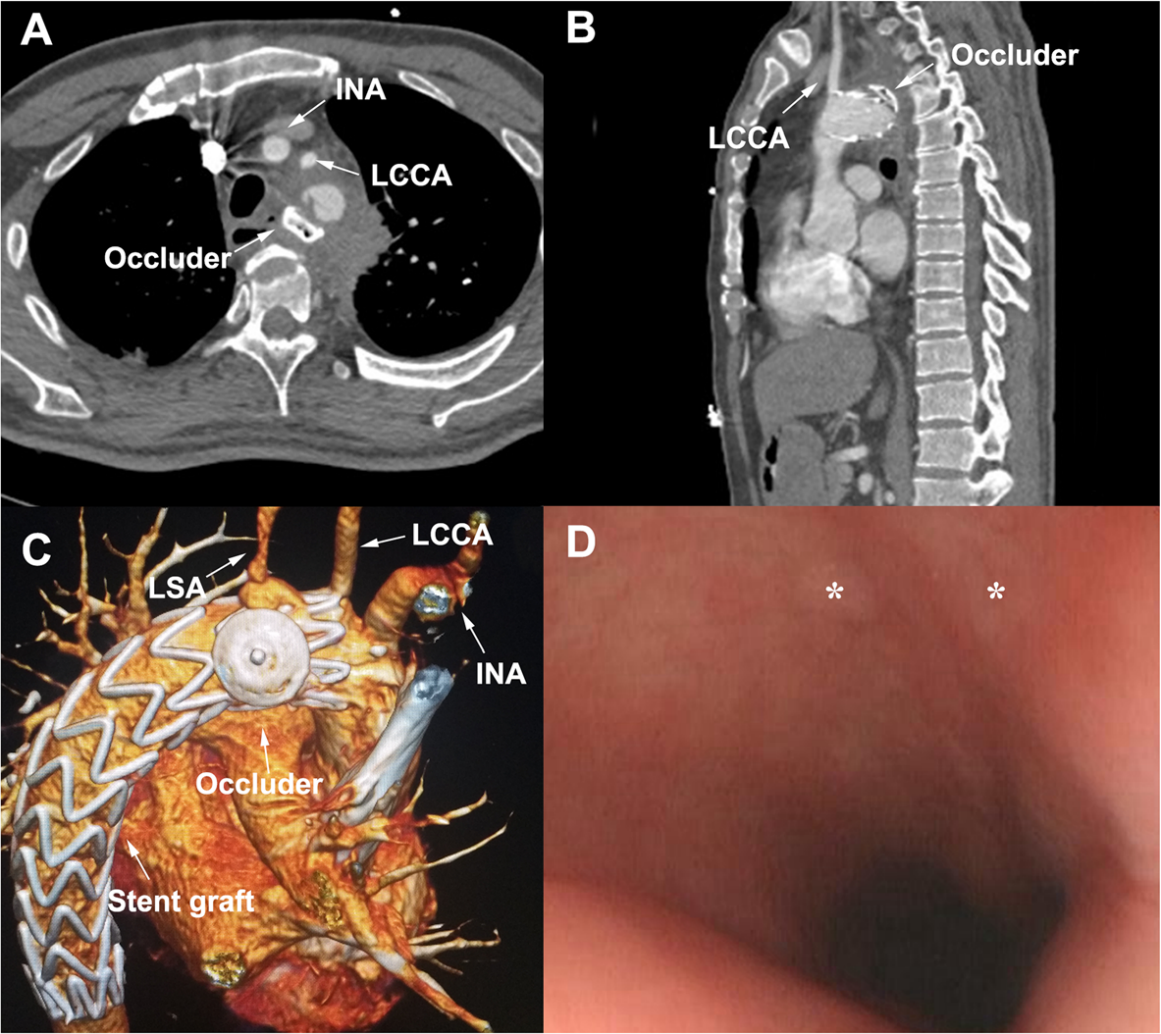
The post-surgical examination: (a) Transverse, (b) sagittal and (c) three-dimensional volume-rendered computed tomography angiography of pre-discharge showed complete sealing of the primary entry tear with the occlude. No endoleak could be found. d Gastroscopic examination revealed the complete healing of original fistulas. *, original esophageal mural ulcers; LCCA, left common carotid artery; LSA, left subclavian artery; INA, innominate artery
